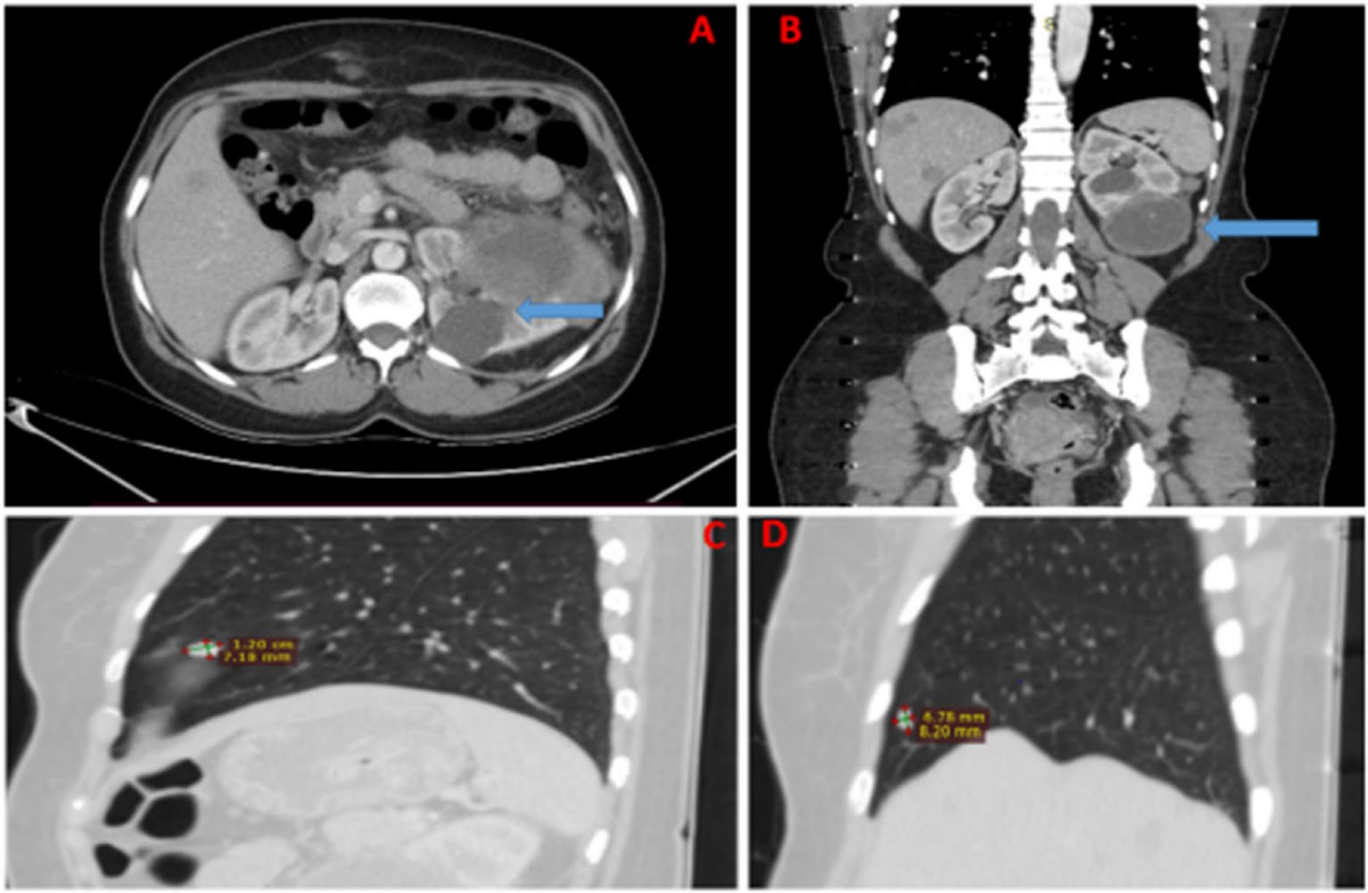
Axial abdominal pelvic CT image (arterial phase) showing a large cortical complex heterogenous enhancing renal mass with cystic and solid components (blue arrow) (A); coronal view of the abdominal pelvic CT scan shows the left renal tumor with solid and cystic components marked on the lower pole. Cysts are seen in the right kidney. Hypodense lesions seen in the liver are suggestive of possible metastasis or hepatic cysts (blue arrow) (B); sagittal CT scan views of the left lung (C) and right lung (D) demonstrate nodules suggestive of metastasis, respectively.
